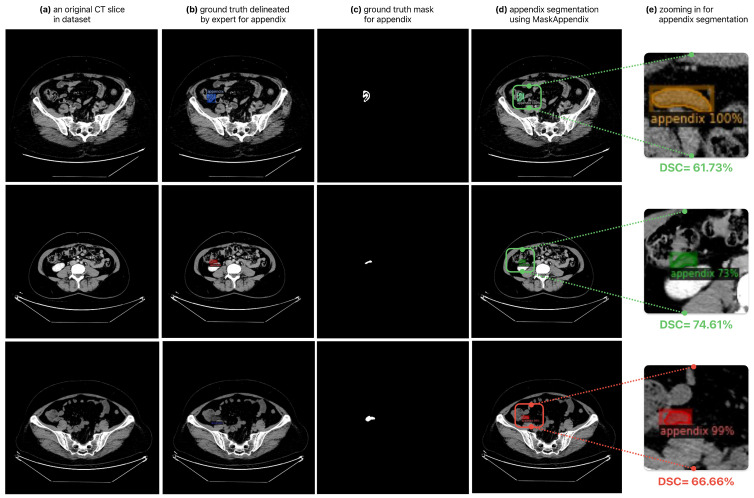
Appendix regions segmented with lower performance using the proposed MaskAppendix model in some CT slices.
